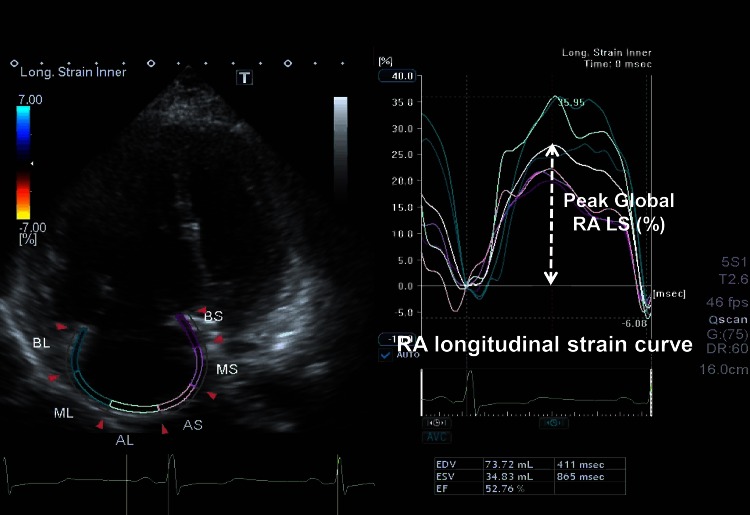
Right atrial longitudinal strain and strain rate determined by 2D speckle tracking echocardiography (2D-STE). The right atrial longitudinal strain and strain rate determined by 2D speckle tracking echocardiography (2D-STE): the right atrial (RA) wall was divided into six segments automatically. Peak right atrial longitudinal strain (RALS) and systolic strain rate (RALSR) were measured in each segment at the mid-RA free wall and interatrial septum, and global values were calculated
